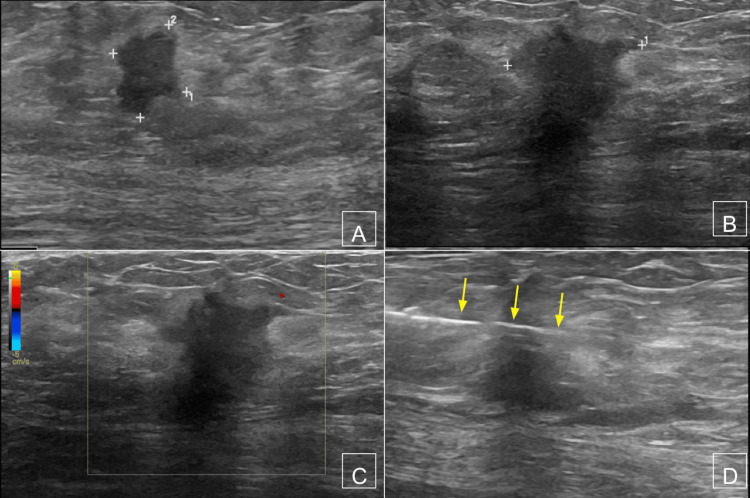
Suspicious right breast mass on sonography (A) Transverse and (B) longitudinal grayscale and (C) sagittal color Doppler images demonstrate 1.9 × 1 × 1.8 cm of spiculated mass in the right breast, corresponding to mammographic mass. An ultrasound-guided biopsy (D) revealed invasive ductal carcinoma (arrows = needle).
